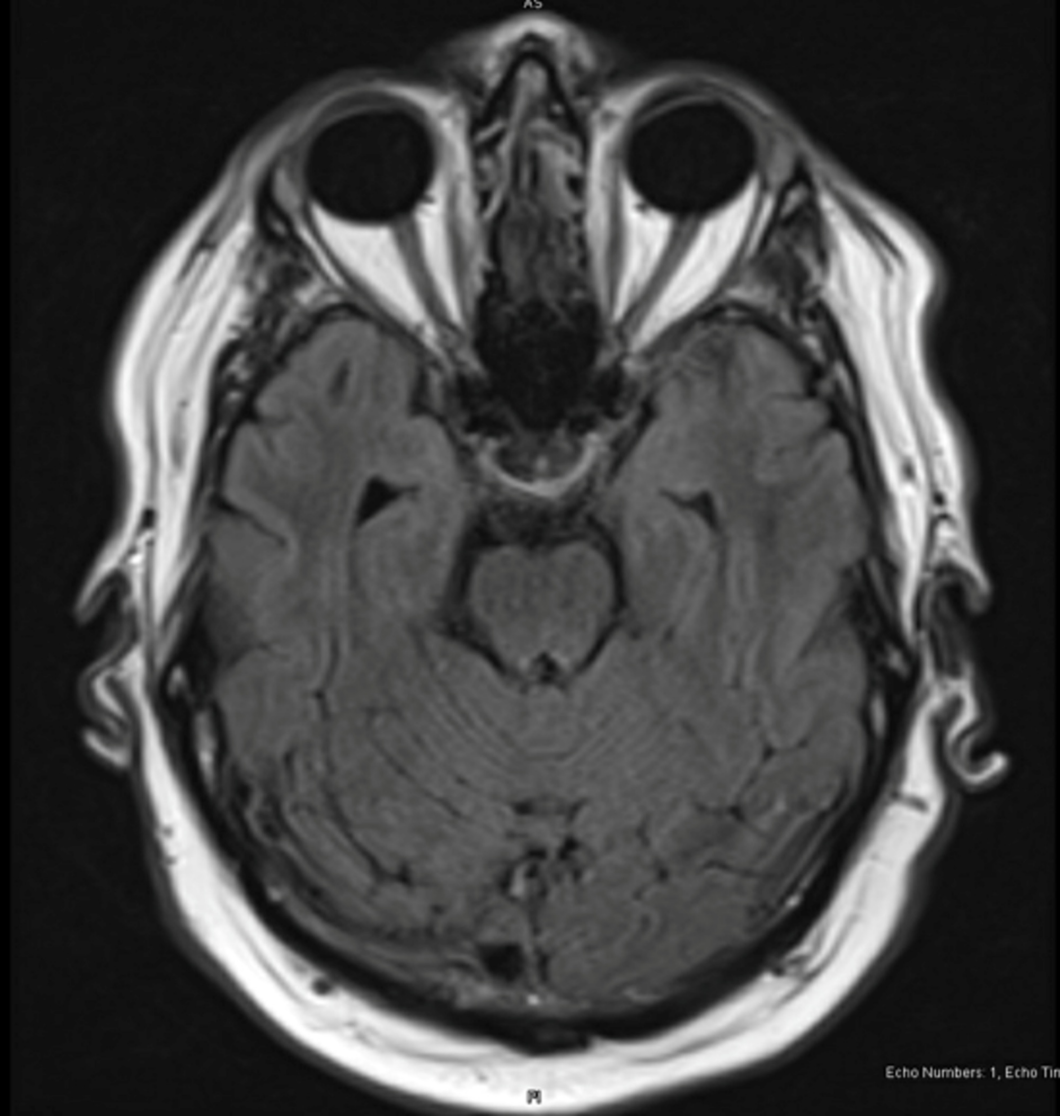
Transverse MRI with no acute findings
